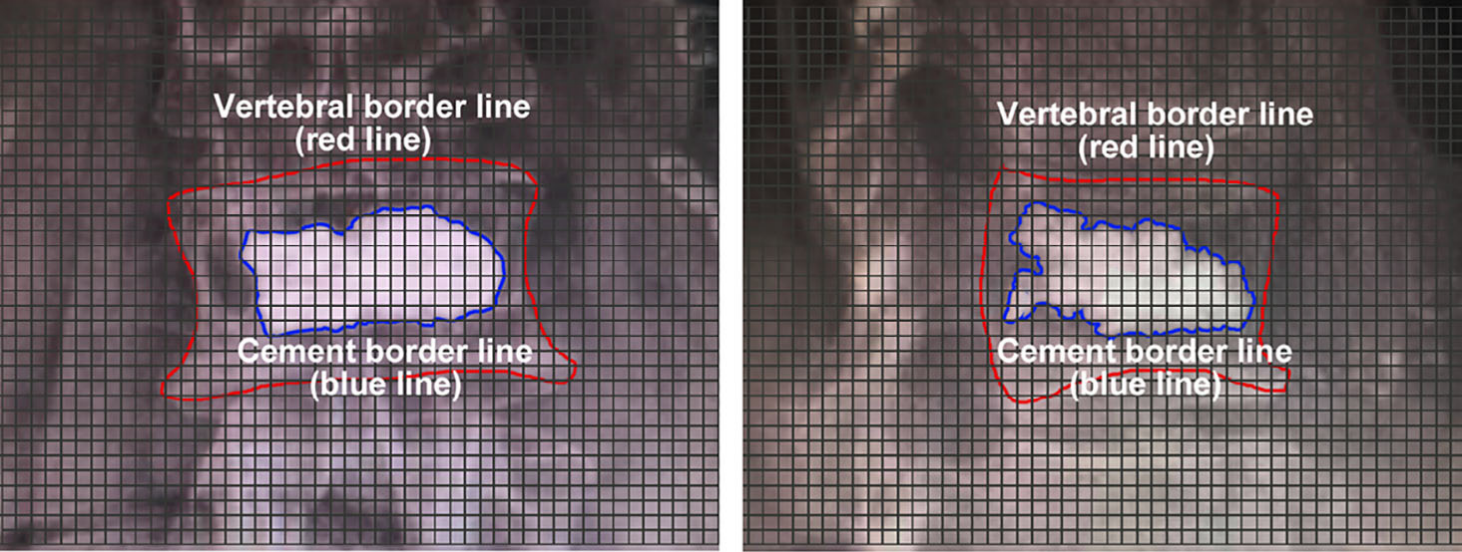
Measurement of cement distribution. According to the anteroposterior and lateral radiographs of the affected spine, the edges of the vertebral bodies are outlined with a red line, and the edges of the bone cement are outlined with a blue line. The entire plane is divided into squares, and the distribution of the bone cement is calculated according to the number of squares occupied by the blue and red lines.
